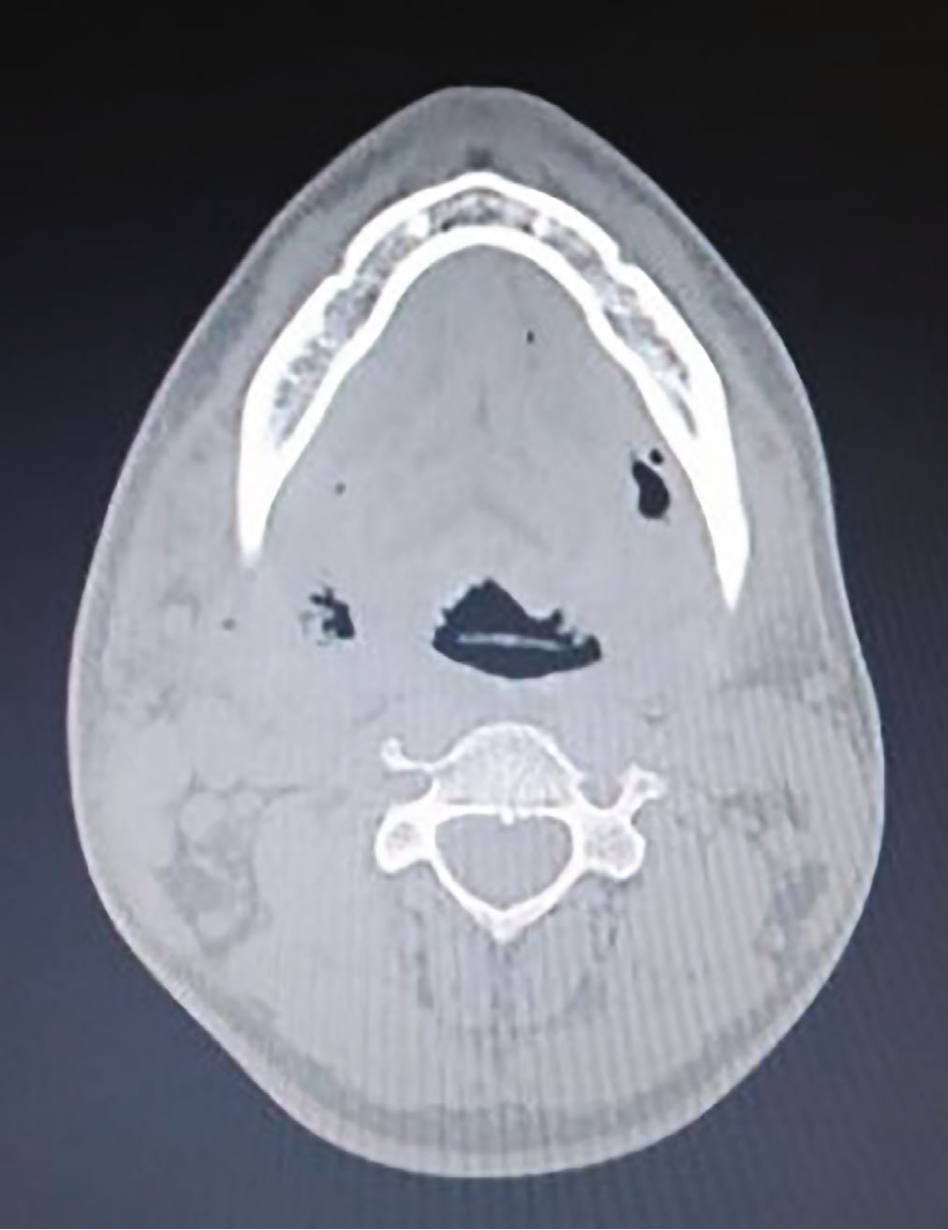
Computed tomography axial view showing the initial aspect of the patient's face.
